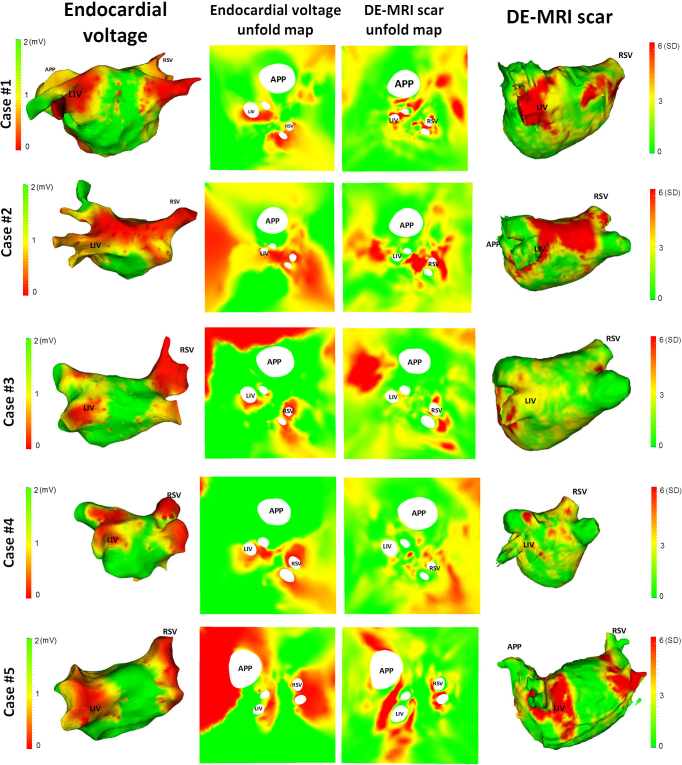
Clinical application II: unfold on 3D electroanatomical LA mesh obtained from mapping systems. Five clinical re-do ablation cases are shown here with electroanatomical map (column 1) and its unfold (column 2) and compared with each case's pre-ablation MRI reconstructed 3D mesh with DE-MRI scar enhancement (column 4) and its unfold (column 3). Note low voltage in milli-volts (mV) is comparable to high intensity in DE-MRI represented as standard deviations (SD) from healthy tissue intensity. Abbreviations for veins – by convention LIV, RSV, APP–appendage. (For interpretation of the references to colour in this figure legend, the reader is referred to the web version of the article.)
